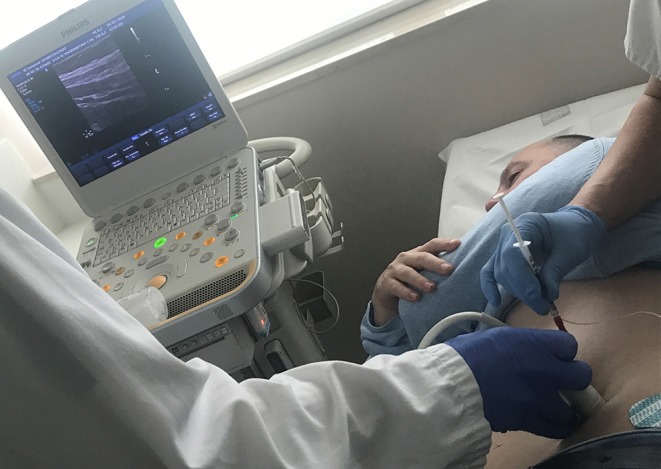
Botulinum toxin application under ultrasonographic and electromyographic guidance on the lateral abdominal wall.
